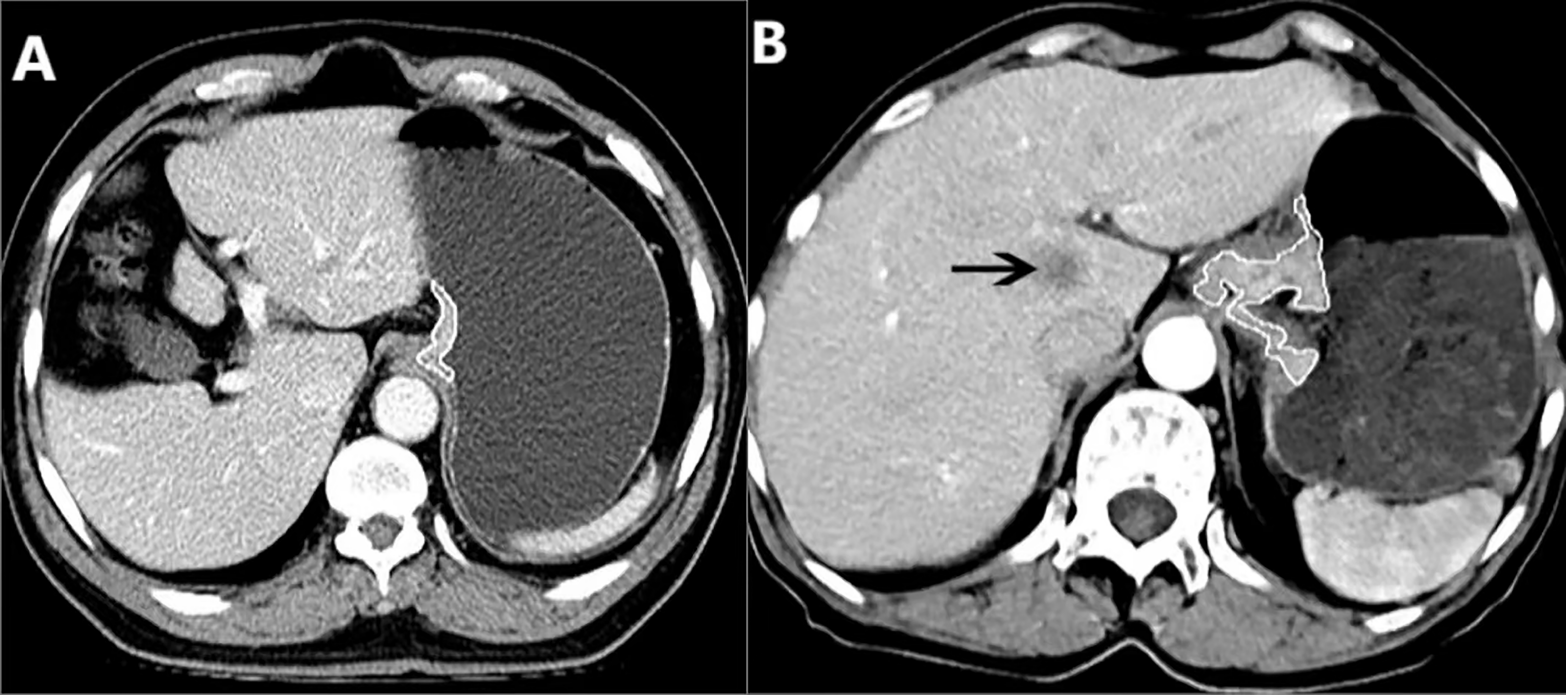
(A) In a 71-year-old male with resectable adenocarcinoma of the esophagogastric junction staged as cT_3_N_1_M_0_, preoperative enhanced CT shows the total tumor volume obtained by manual sketching layer by layer along the edge of abnormal distal esophageal and proximal gastric wall, and the total tumor volume is 10.20 cm^3^. (B) In a 70-year-old female with unresectable adenocarcinoma of the esophagogastric junction staged as cT_3_N_1_M_1_, the enhanced CT shows the metastasis in the liver (arrow), and the total volume of the tumor is measured by manual sketching layer by layer along the edge of the thickened distal esophageal and proximal gastric wall, and the total volume of the tumor is 35.62 cm^3^.
